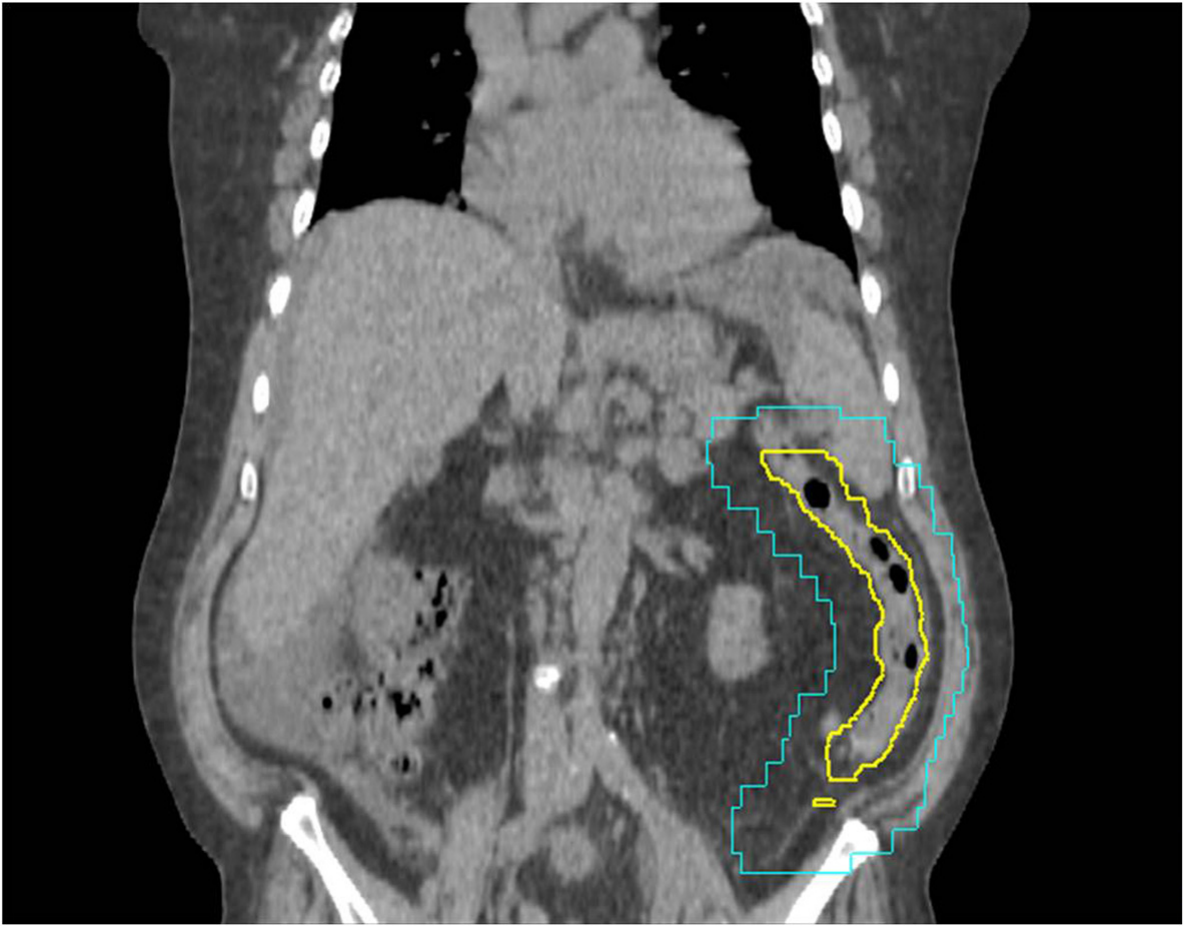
Computed tomography of palliative radiotherapy plan without clips. Representative coronal CT image of the CTV (yellow) and radiation field edges (blue) without fiducial markers present. No GTV was visible therefore the CTV contour included the entire descending colon.
